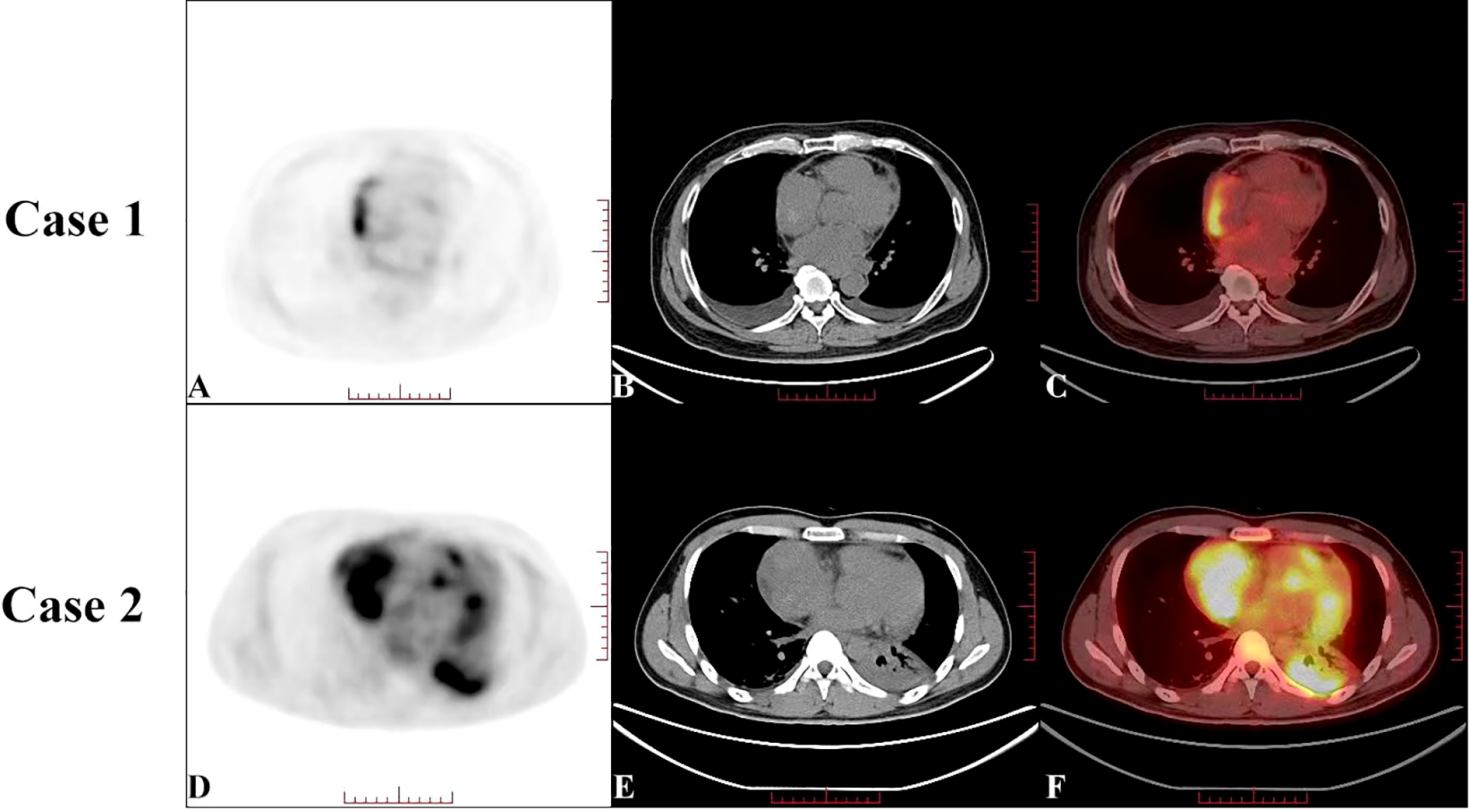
Preoperative positron emission tomography-computed tomography (PET-CT) of Case 1 (A–C) showed an increase in radiotracer uptake in an arcuate-shaped area in the outer wall of the right atrium, with a maximum standard uptake value (*SUV*
